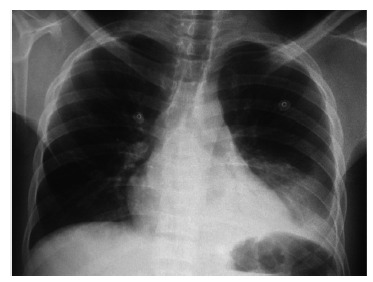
Chest X-ray demonstrate left lower lobe opacification with minimal pleural effusion in a 16 years sickle cell disease patient presented with acute chest syndrome. The patient improved markedly after exchange blood transfusion.
